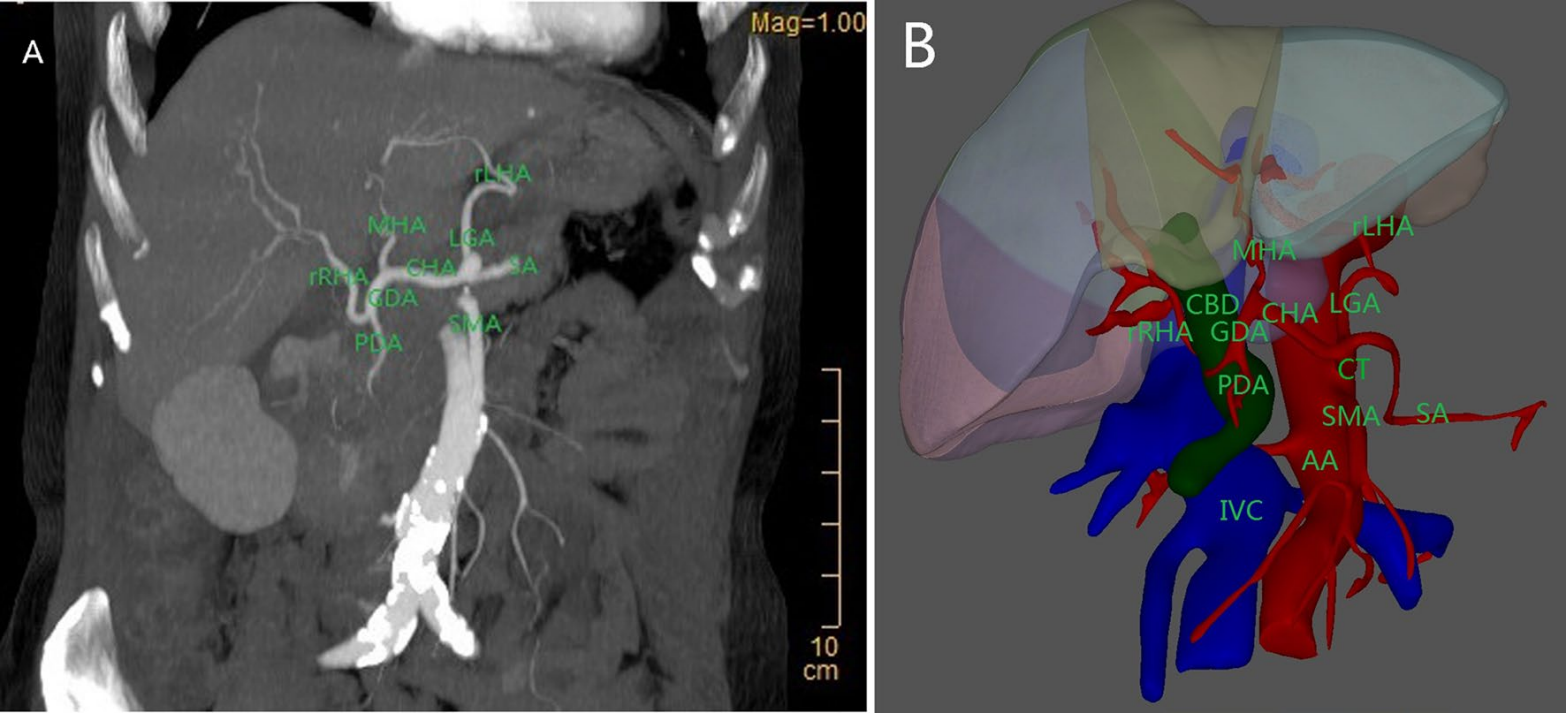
Coronal maximum intensity projection (MIP) and three-dimensional reconstruction images based on preoperative contrast-enhanced CT. A MIP image shows that the rRHA arose from the GDA, entered into the right liver lobe; MHA was derived from the CHA, and rLHA was branched from the LGA. B Three-dimensional reconstruction shows the spatial anatomical relationship of the liver, artery, and CBD; the rRHA traversed anterior to the CBD and supplied right liver lobe; the MHA extended to porta hepatis was erroneously identified as PHA intraoperatively, which entered the liver substance at the fissure between the right and left lobes, and actually supplied hepatic segment IV. *rRHA* replaced right hepatic artery, *rLHA* replaced left hepatic artery, *MHA* middle hepatic artery, *GDA* gastroduodenal artery, *LGA* left gastric artery, *SA* splenic artery, *PDA* pancreaticoduodenal artery, *CHA* common hepatic artery, *SMA* superior mesenteric artery, *CBD* common bile duct, *AA* abdominal aorta, *IVC* inferior vena cava, *CT* celiac trunk
